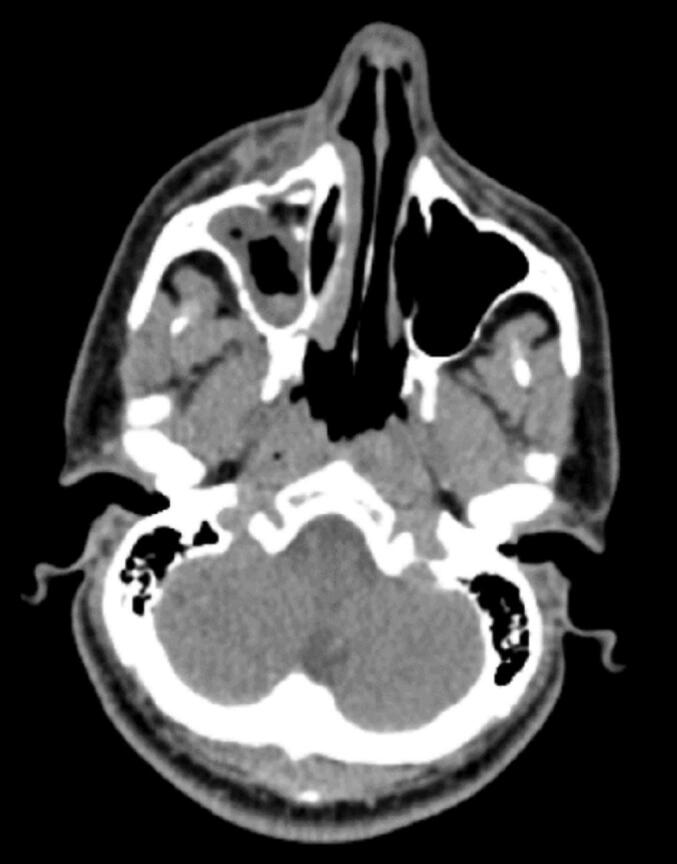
CT of Right Maxillary Sinus involvement by Tumor.
